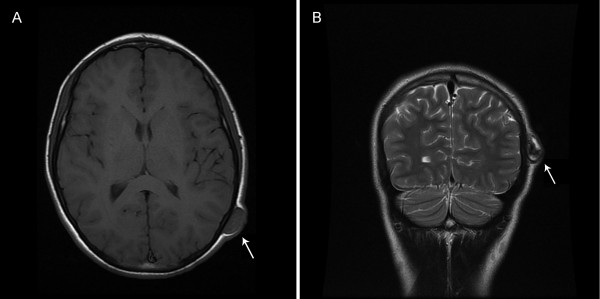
Preoperative T1-weighted axial and T2-weighted coronal magnetic resonance imaging. (A) Preoperative T1-weighted axial magnetic resonance imaging and (B) T2-weighted coronal magnetic resonance imaging shows an evolving hematoma (arrow) in the vicinity of the occipital artery.
